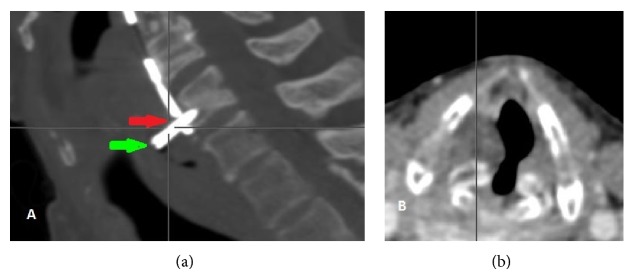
Sagittal reconstruction of the neck CT shows an extrusion of the screw at the C6 level (red arrow) and damaged postcricoid mucosa (green arrow) (a). 3D reconstruction of the plate and screws. 11 mm back-out of the one screw is noticeable (b).
